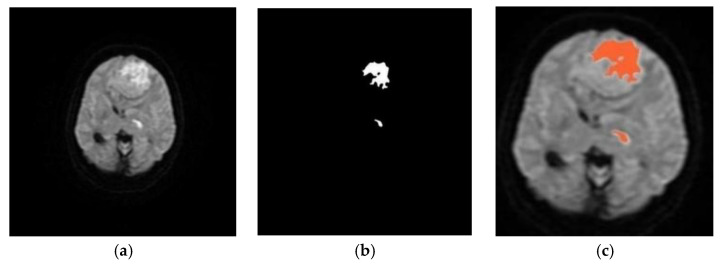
Original DWI image (a), and morphological binary image (b), detected infarct marked as red (c) [78].
